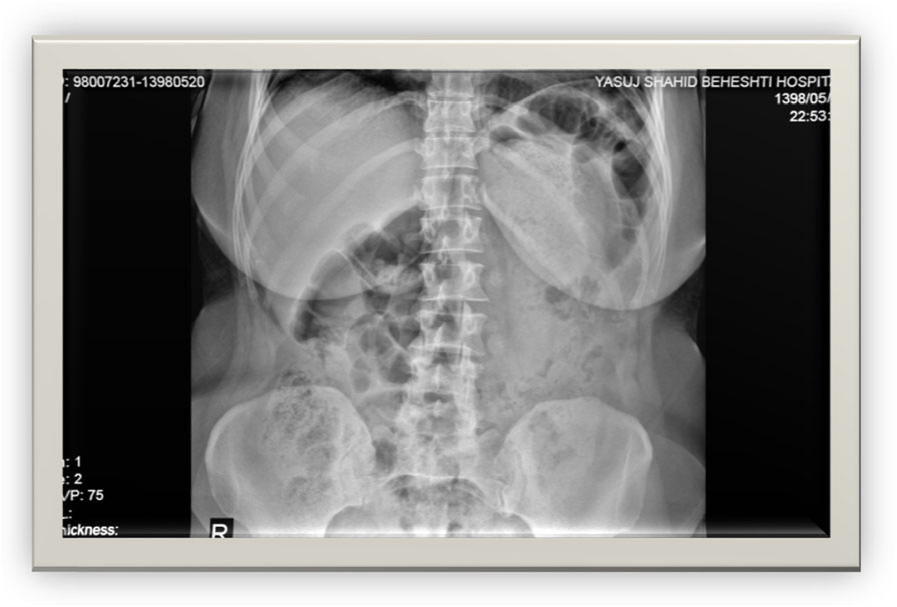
Normal upright abdominal x-ray
